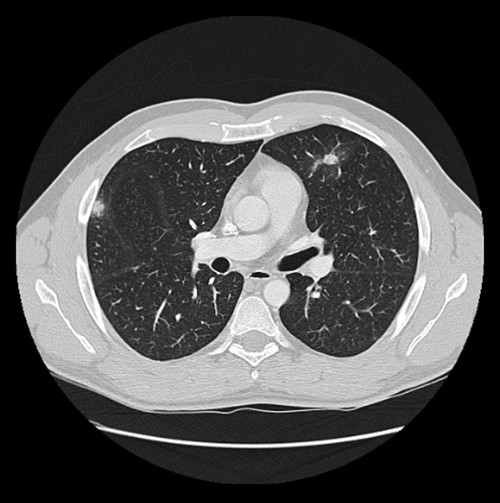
Computed tomography: macro nodules with ground-glass halo.
